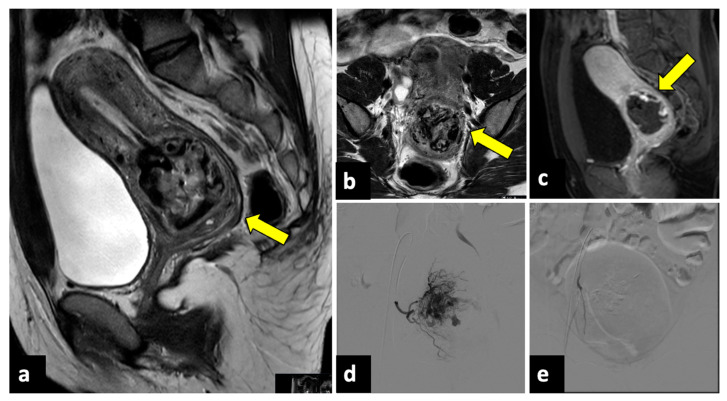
Ruptured cervical ectopic pregnancy in a thirty-three-year-old patient who presented at the emergency department with acute pelvic pain and severe vaginal bleeding. MRI sagittal (a) and axial (b) T2-weighted, and sagittal T1-weighted early contrast-enhanced fat-saturated (c) images show an empty uterine cavity and a ruptured ectopic pregnancy within the cervical canal (yellow arrows). This patient was managed with uterine arteries embolisation. Preprocedural images (d) show a hypervascular area in the region of the cervical ectopic pregnancy that is supplied mainly by the right uterine artery. Post-embolisation (e) arteriogram demonstrates complete stasis.
